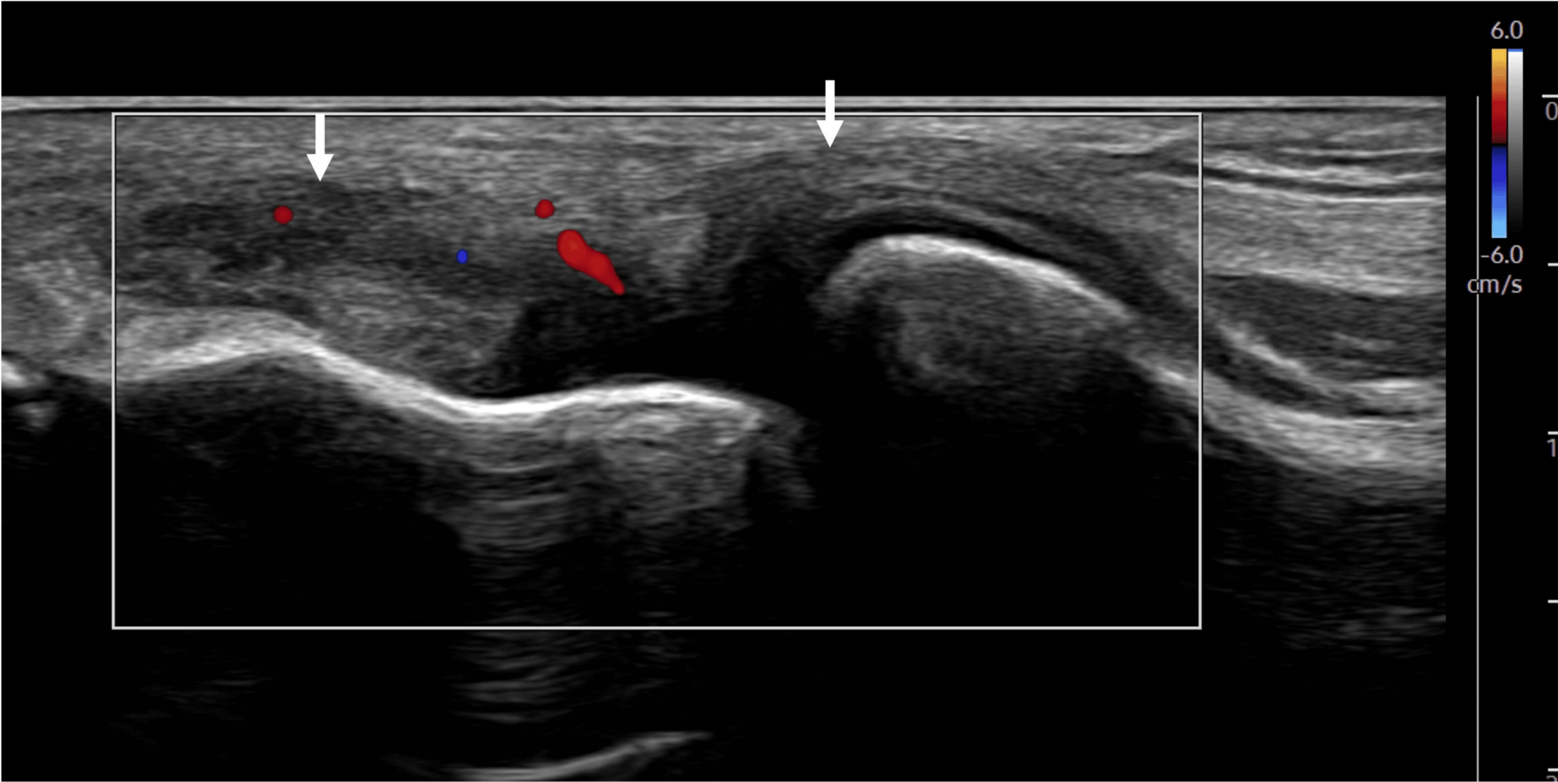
Long-axis view of the common extensor tendon with the color Doppler tool showing mild hyperemia within the tendon. Arrows: Common extensor tendon.
